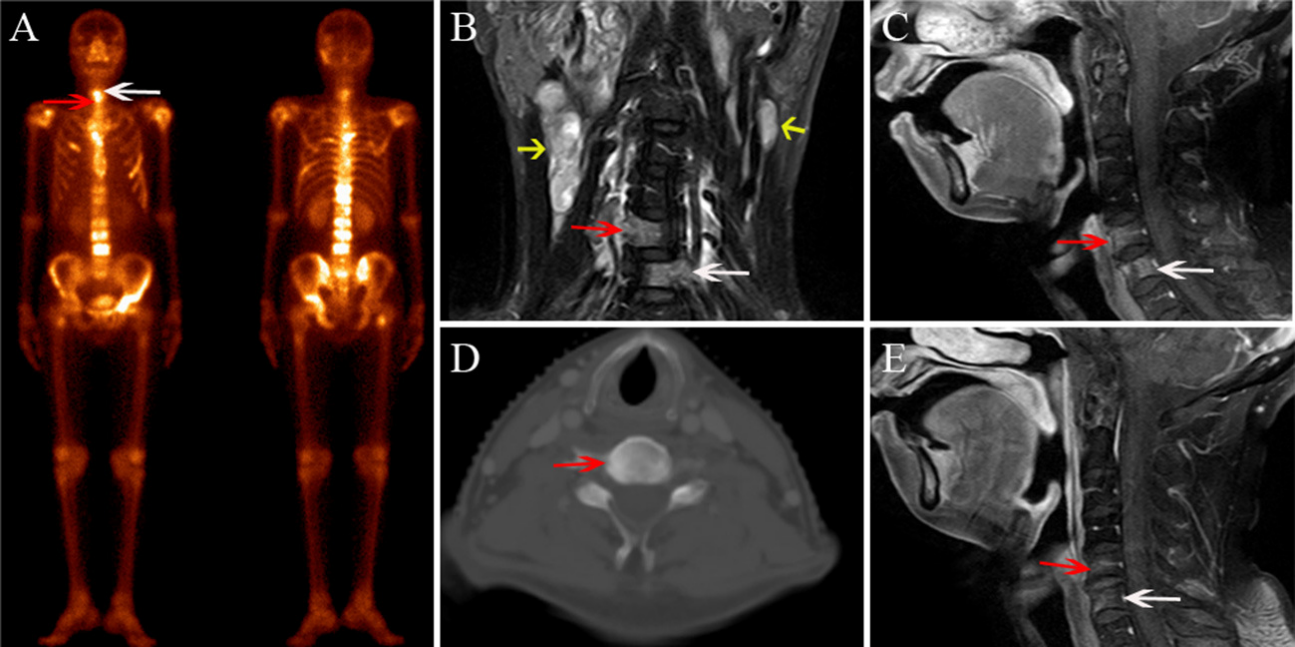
A 68-year-old female diagnosed with bone metastasis after radiotherapy for NPC. (A) BS shows increased radiotracer uptake in C5 (white arrow) and C6 (red arrow); multiple ribs, thoracic vertebrae, lumbar vertebrae, and the right femoral neck also show increased radiotracer uptake. (B) Coronal FS T2-weighted image shows hyperintensity in C5 (white arrow) and C6 (red arrow), and multiple enlarged lymph nodes in both sides of the neck (yellow arrow). (C) Sagittal contrast-enhanced T1-weighted image shows marked and heterogeneous enhancement in C5 and C6. (D) CT localization image shows an osteogenic change in C5. (E) In the MRI follow-up 2 months after RT, the sagittal contrast-enhanced T1-weighted image shows that the area of the lesions has shrunken and the enhancement has declined.
